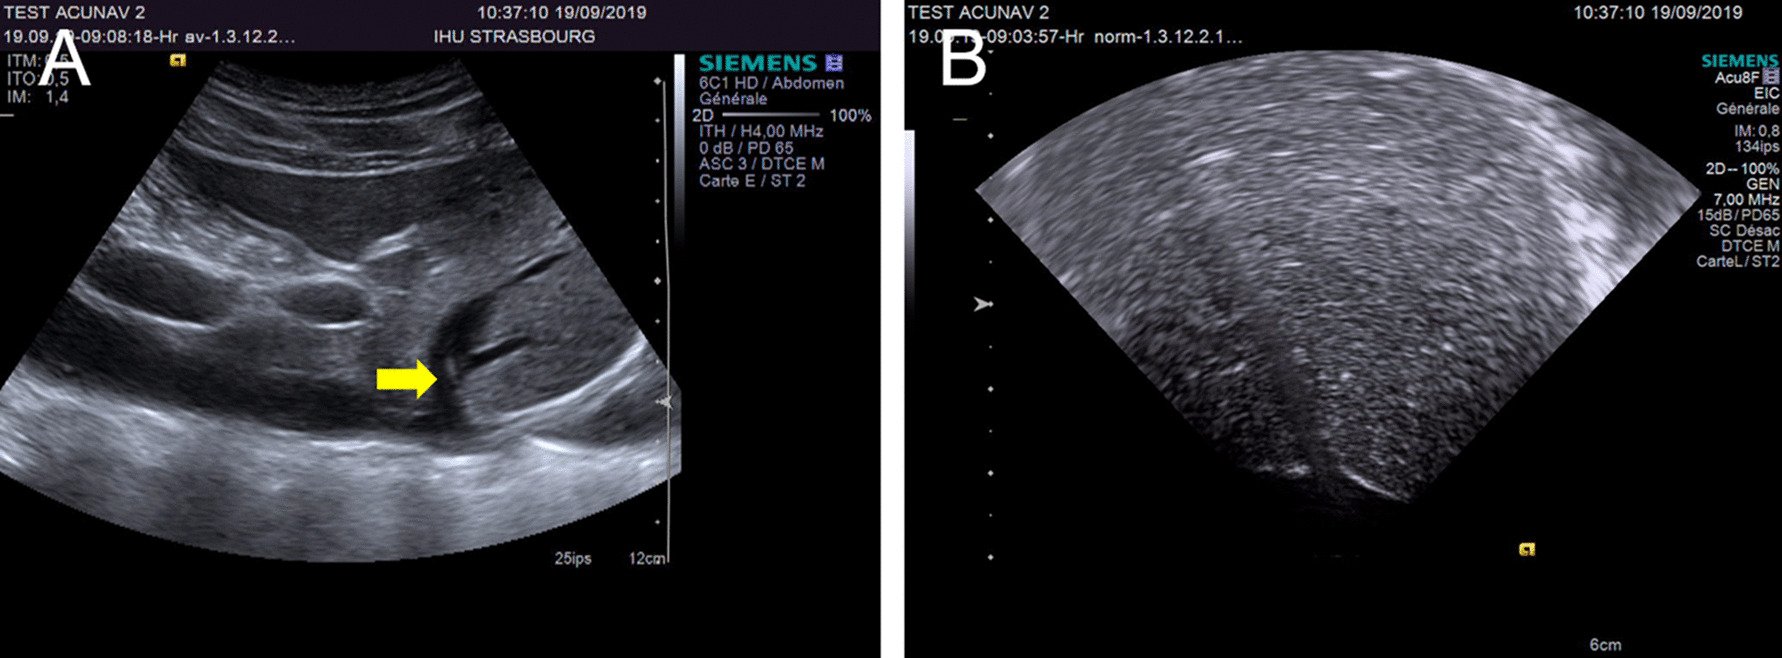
External ultrasound enabled confirmation of the location of the intravascular catheter. a External ultrasound detected the intravascular catheter tip (yellow arrow) in the left median hepatic vein. The right side of the image is cranial and the left one is caudal. b Intravascular ultrasound image from the left median hepatic vein. The right side of the image is proximal and the left one is distal
